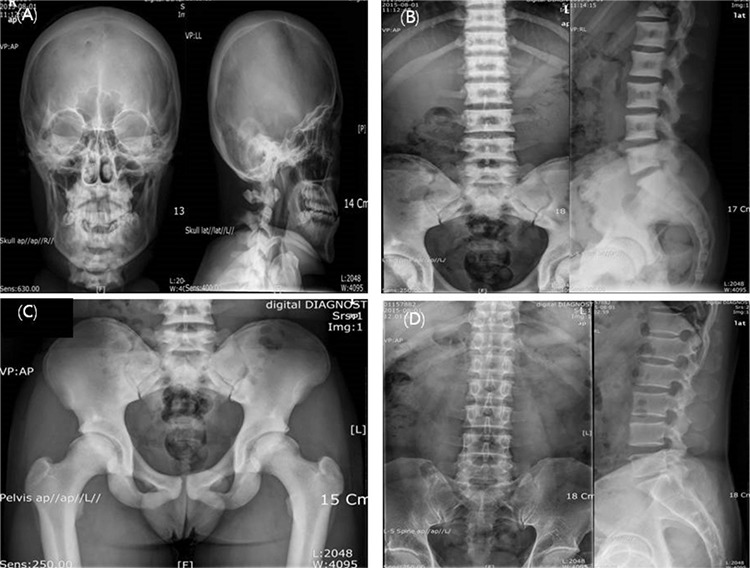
A) X-rays of the skull showing generalized increase in bone density. The sclerosis is more prominent in the base of the skull. B) Typical end-plate thickening and sclerosis producing the classic “sandwich vertebrae” appearance. C) Sclerosis in the iliac wings, acetabuli and femur heads. However, typical “bone-within-bone” appearance cannot be noted in the patient. D) The patient’s father showed normal bone density
